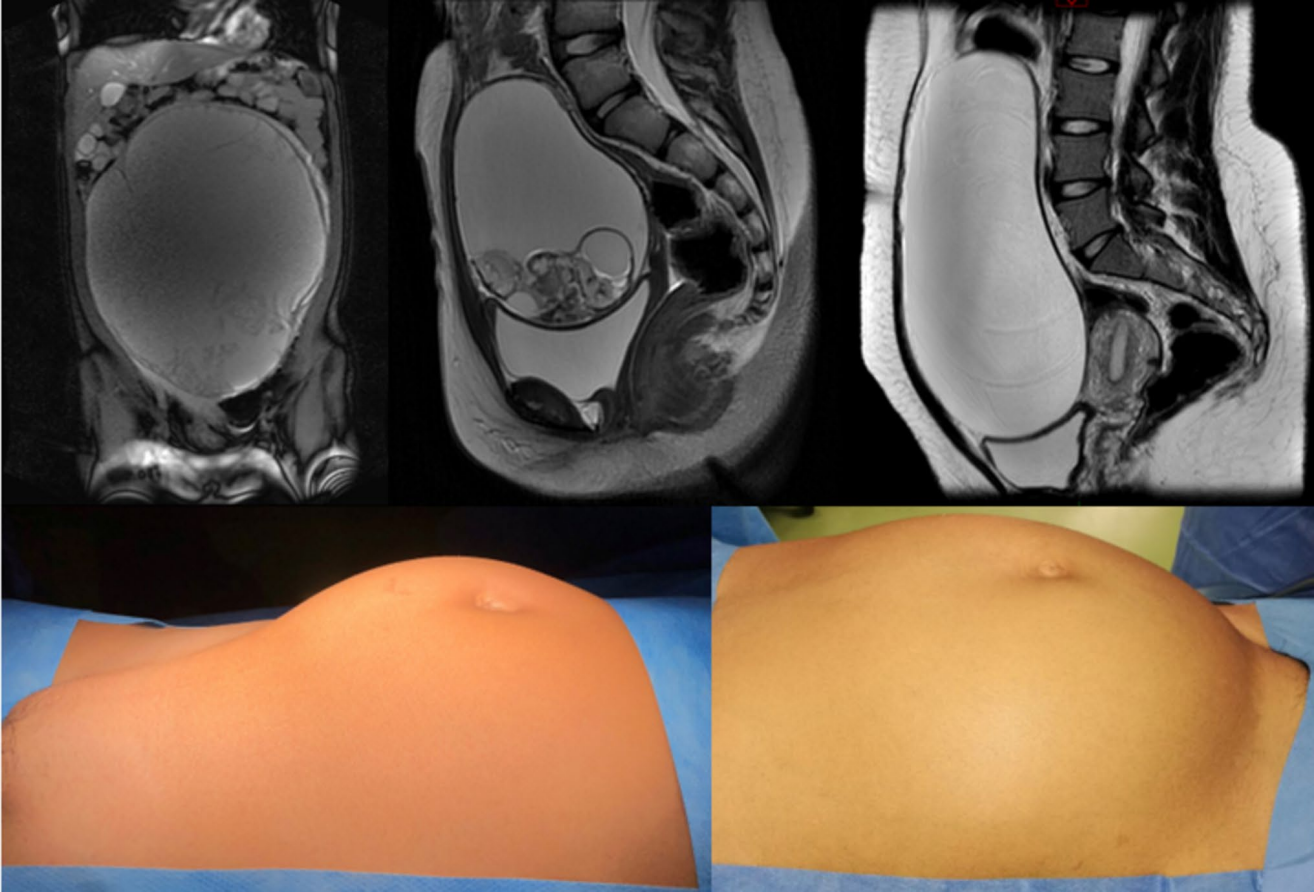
Imaging and physical characteristics of representative cases. (Top panel) MRI appearance of the large ovarian tumor of case #1,2 and 4, respectively. Note the predominantly cystic pattern of the lesions. (Bottom panel) Appearance of the remarkably distended abdomen of the smallest and largest cystic ovarian tumor of present series (case #2 and 1, respectively)
